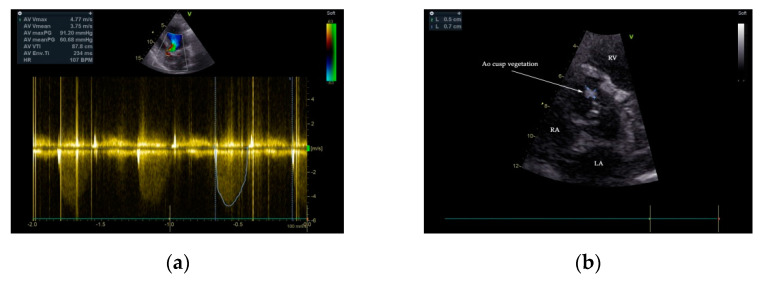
TTE 2D color flow and continuous Doppler 2D examination demonstrated severe aortic stenosis—aortic valve gradient 91/60 mmHg (a). The presence of a vegetation of 5 mm × 7 mm attached to the anterior cusp of the aortic valve is depicted (b).
